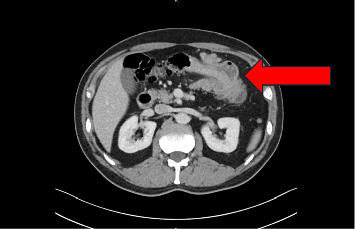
Axial CT showing colocolic intussusception in the left hemiabdomen.
